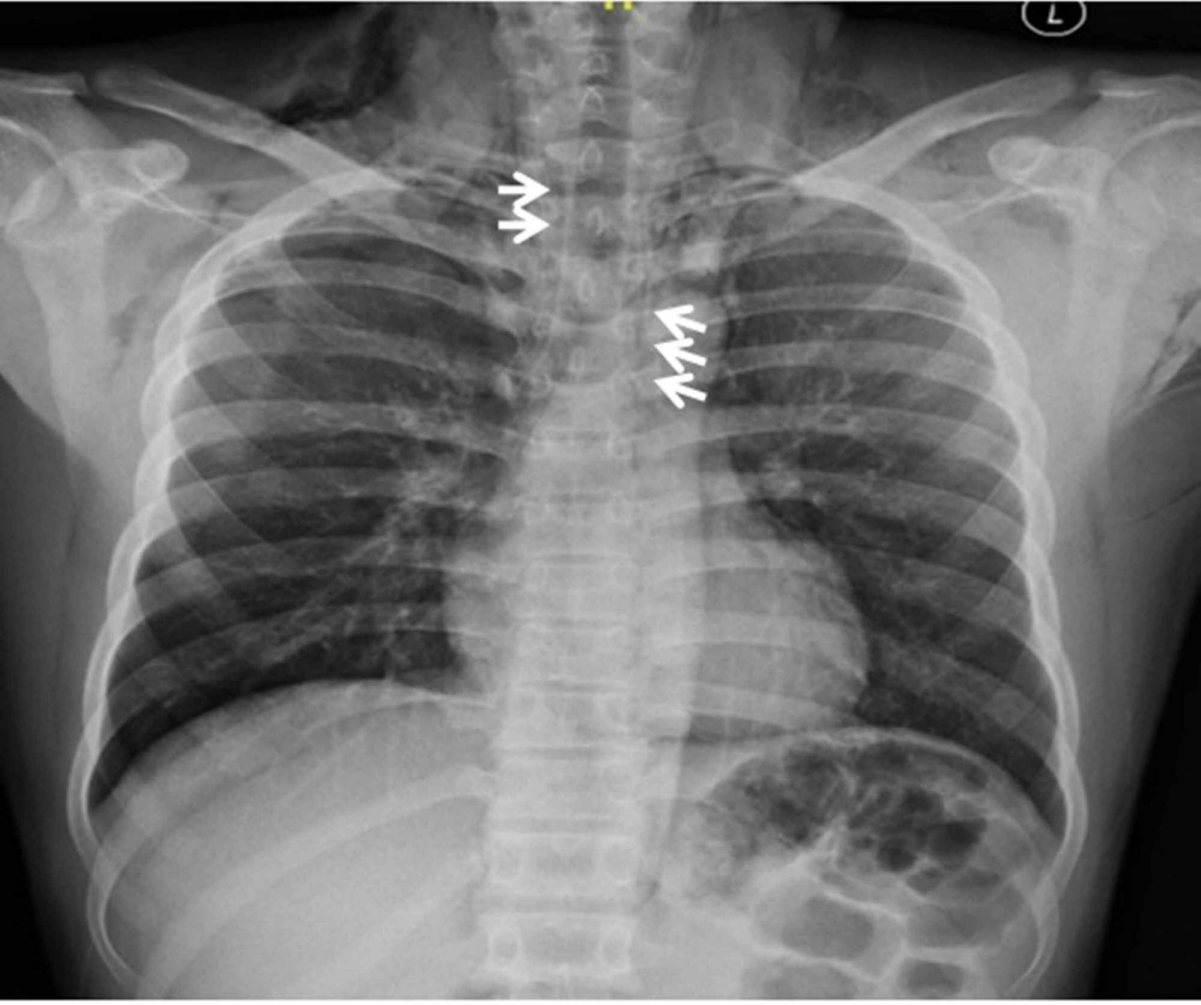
X-ray chest showing pneumomediastinum (arrows)
